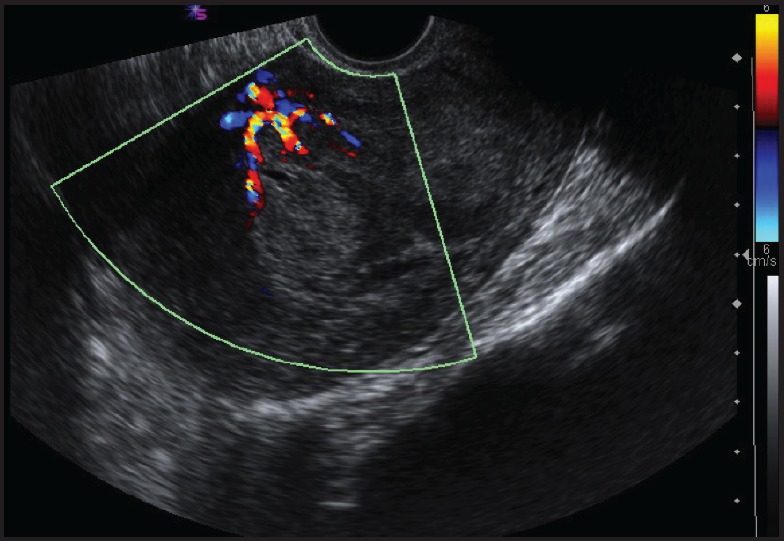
Transvaginal ultrasound described an enlarged uterus with endometrial thickening, confined to endometrial cavity. Important vascularisation detail, by applying colour Doppler.
